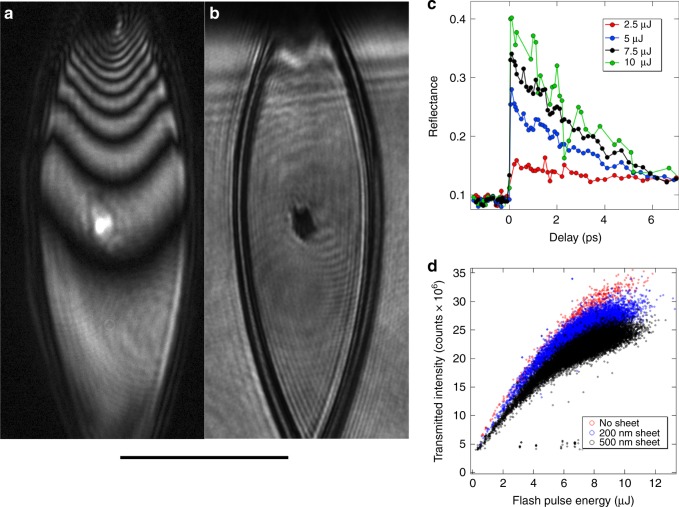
Soft X-ray measurements of liquid water sheets. Measurements from the FLASH free-electron laser are shown as described in the text. Shown are optical reflection (a) and transmission (b) images taken in situ. The entire sheet was illuminated by an optical parametric amplifier (500 nm), which was used as a probe of the dynamics induced by the soft X-ray pulses. The X-ray spot can be seen as a bright spot in reflection and a dark spot in transmission, immediately following the X-ray pump. c The reflectivity of the X-ray pumped region of the water sheet, as a function of time delay between the X-ray pump and optical probe, for a series of different pump fluences. d The intensity of soft X-rays transmitted through the water sheet, as a function of FLASH pulse energy, for different sheet thicknesses. The scale bar is 100 μm
